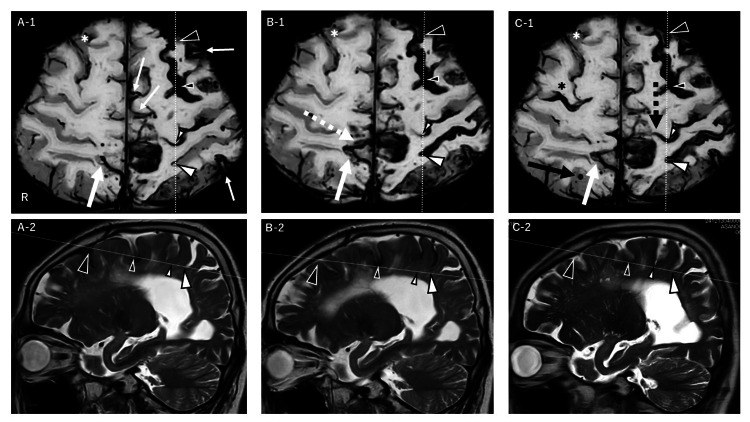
Serial axial SWI and sagittal T2WI MRI showing CSAH and CSS progression A-1, A-2: Four months post-initial visit, SWI shows linear hypointensities consistent with cSAH in left frontal sulcus. A hypointense line is noted in right frontal lobe, with discontinuous or double-line signals in right superior frontal sulcus. The left postcentral sulcus shows a thin hypointensity; left hemisphere demonstrates typical cSS (small white arrows). The T2-sagittal images show marked low signal in the anterior left frontal sulcus, milder posteriorly. The central sulcus (small white arrowhead) has hypointensity in the sulcal space and superficial cortex; the postcentral sulcus is hyperintense. B-1, B-2: Seven months after initial visit, the anterior left superior frontal sulcus evolves into blurred double-line hypointensity; posterior changes are minimal. New cSAH appears in left postcentral sulcus and right parietal lobe (white dotted arrow). The T2WI shows reduced anterior sulcal signal. The central sulcus appears narrowed with faint residual hypointensity; low-signal core emerges in postcentral sulcus. C-1, C-2: 10 months after initial visit, SWI shows a clear double-line in anterior left sulcus; posterior remains stable. New cSAH is seen in right superior frontal sulcus. The central sulcus shows medial hypointense extension (black dotted arrow); left postcentral sulcus shows cSS. The T2WI shows anterior low signals and resolved; posterior ones persist. The central sulcus widens with hyperintensity and subtle residual low signal. Black arrowhead: Anterior left superior frontal sulcus; Small black arrowhead: Posterior segment; White arrowhead: Left postcentral sulcus; Small white arrowhead: Left central sulcus; White asterisk: Right superior frontal sulcus; White arrow: Hemorrhagic focus in the right parietal lobe; Vertical dotted line: Sagittal plane (A-2 to C-2); Oblique dotted line: Axial plane (A-1 to C-1) SWI: Susceptibility-weighted imaging; T2WI: T2-weighted imaging; cSAH: Convexity subarachnoid hemorrhage; cSS: Cortical superficial siderosis
